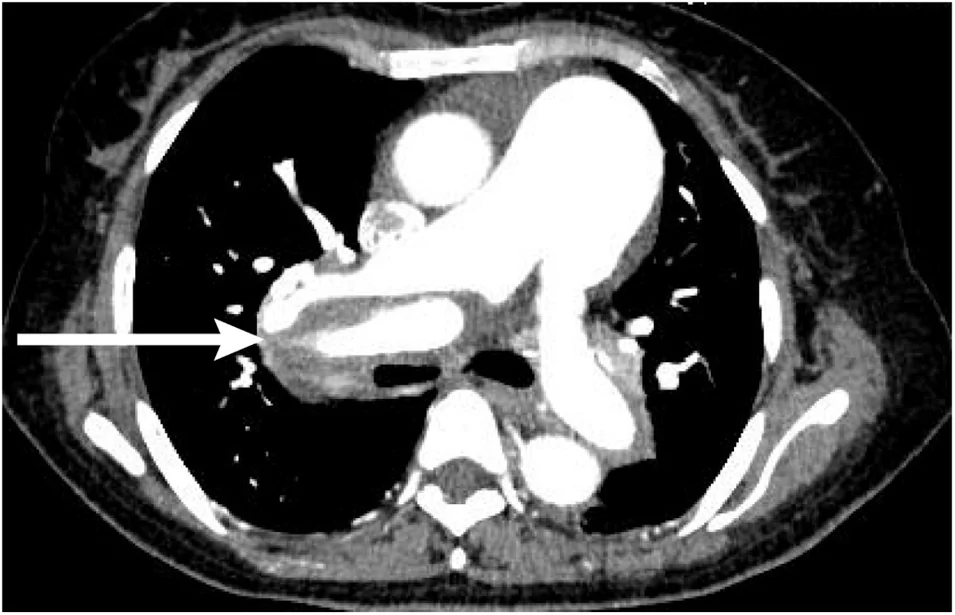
Enhanced CT revealed the right-side pulmonary artery dissection involved the pulmonary trunk and the right pulmonary artery (arrow).
